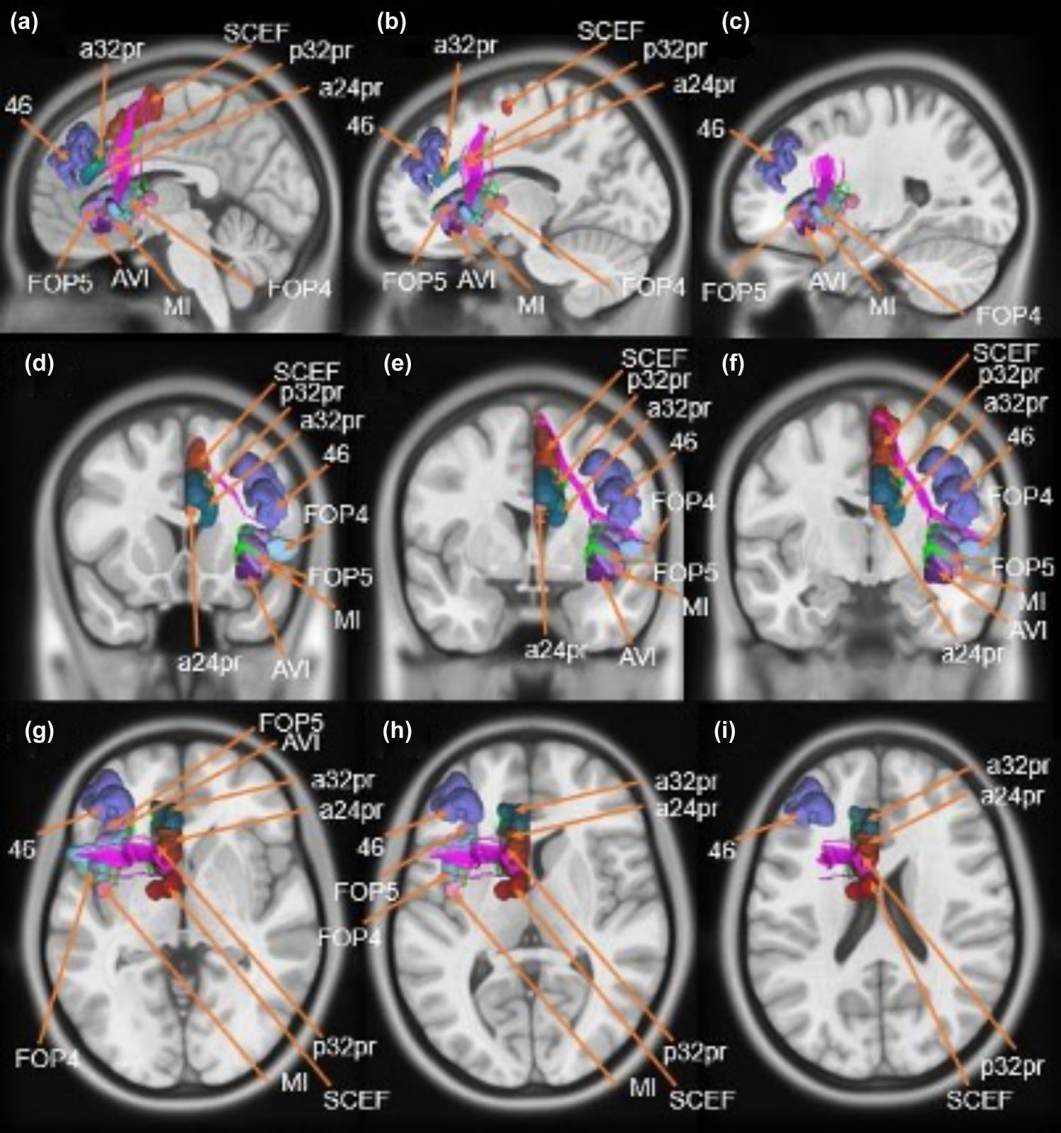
Fiber tracking analysis for the salience network (SN). T1‐weighted MR images in the left cerebral hemisphere are shown. Top row: sagittal sections from most posterior to most anterior demonstrating the frontal aslant tract (FAT) and its projections between the opercular, insular, and middle cingulate clusters of the SN. Middle row: coronal sections from medial to lateral through the parietal and occipital clusters demonstrate the FAT and the short fiber connections within the network. Bottom row: axial sections from inferior to superior provide another view of the FAT and short fiber connections within the network. Abbreviations: a24pr, anterior 24 prime; a32pr, anterior 32 prime; AVI, areas anterior ventral insula; FOP4, frontal operculum 4; FOP5, frontal operculum 5; MI, middle insula; p32pr, posterior 32 prime; SCEF, supplementary and cingulate eye field
